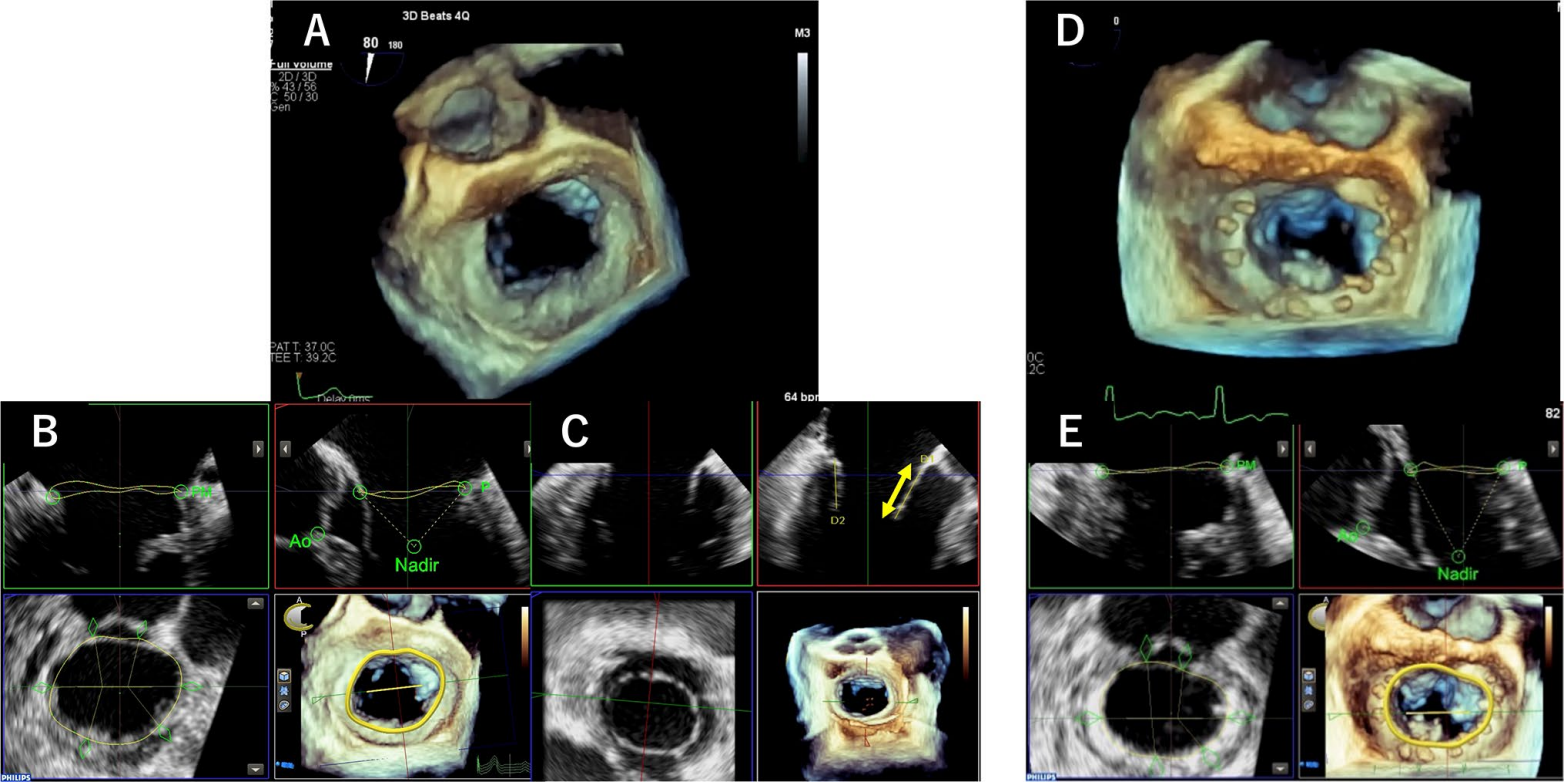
Methods of three-dimensional analysis of mitral valve using transesophageal echocardiography. From preoperative three-dimensional transesophageal echocardiographic (3D TEE) data (A), the mitral annulus area, circumference diameter (yellow circle), lateral diameter (pink arrow), and anterior to posterior diameter (blue arrow) of the mitral annulus were measured using MVN software at mid-diastolic phase (B). A two-dimensional long-axis view perpendicular to the anterior (A2)–posterior (P2) coaptation line was reconstructed, and the lengths of A2 (white arrow) were measured (C). From postoperative 3D TEE data (D), the mitral annulus area was measured using MVN software as well as preoperative analysis at the mid-diastolic phase (E)
